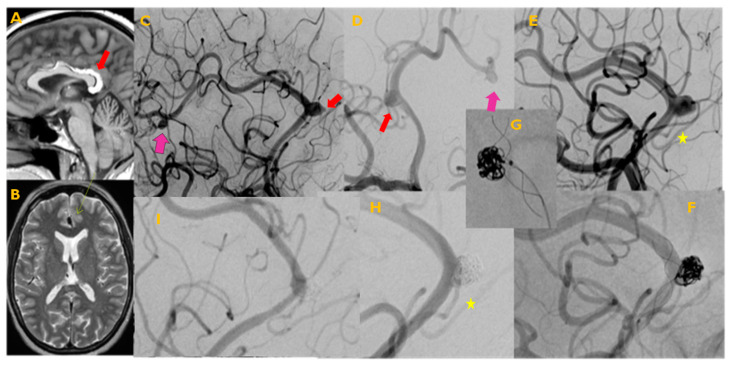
Patient in their late sixties, initial MRI performed for atypical headaches, absence of known cardiovascular risk factors. (A) FLAIR, sagittal plane, incidental finding of corpus callosum lipoma (red thick arrow); (B) axial T2 suspicion of IA over a signal void adjacent to the lipoma, (thin arrow); (C) digital subtraction angiography, lateral plane, showing the suspected pericallosal A3 aneurysm (small, thick arrow), with a prominent phlyctena at the dome, as well as a second, A4 aneurysm (big arrow); (D) oblique plane showing the same aneurysms, both with cortical branches coming out of the sacs; (E) working projection, asterisk, branch coming out of the aneurysm’s sac; (F,G) working projection, unsubtracted image showing stent-assisted coiling, with a stent LEO Baby 3 × 18 mm (Balt extrusion, Montmorency, France) and coils (Stryker, Kalamazoo, MI, USA); (H) angiographic result at the end of the procedure showing good wall apposition of the stent, occlusion of the aneurysm, and excellent permeability of the jailed branch (yellow asterisk); (I) DSA control at 6 months, showing occlusion of the aneurysm and complete patency of the jailed branch.
